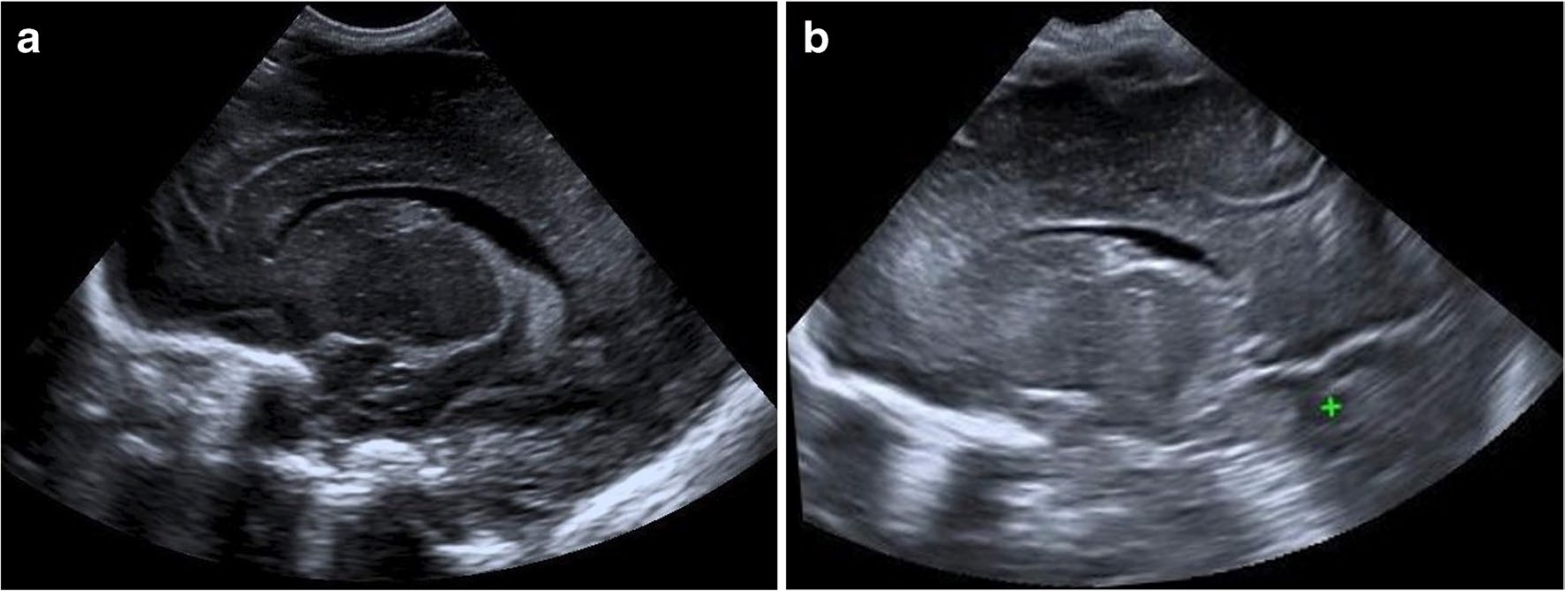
An 18-day-old girl with a normal cranial ultrasound (US). a Two-dimensional (D) US sagittal view of the right caudothalamic groove. One observer rated this view quality as ‘good’, and two ‘adequate’. b Sagittal reconstruction of the 3-D volumetric US dataset of the right caudothalamic groove view. Note the pivot point used for 3-D manipulation and reconstruction (*caliper*). The image also demonstrates the smaller near field view of the 3-D acquisition. One observer rated this view quality as ‘good’, one ‘adequate’, and one ‘poor’
